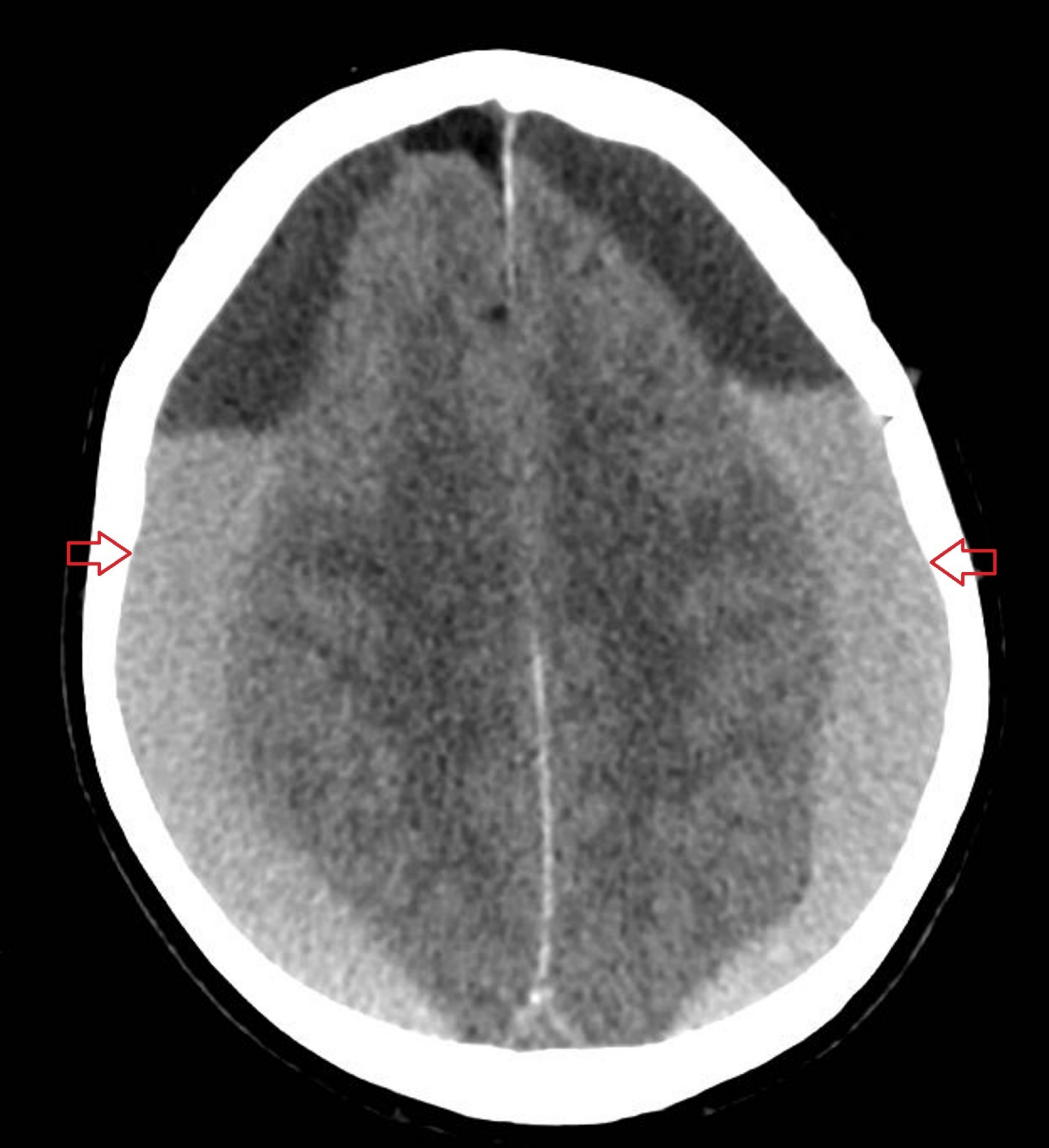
Axial non-contrast computed tomography scan of the brain Showing bilateral acute-on-chronic subdural haemorrhages.
